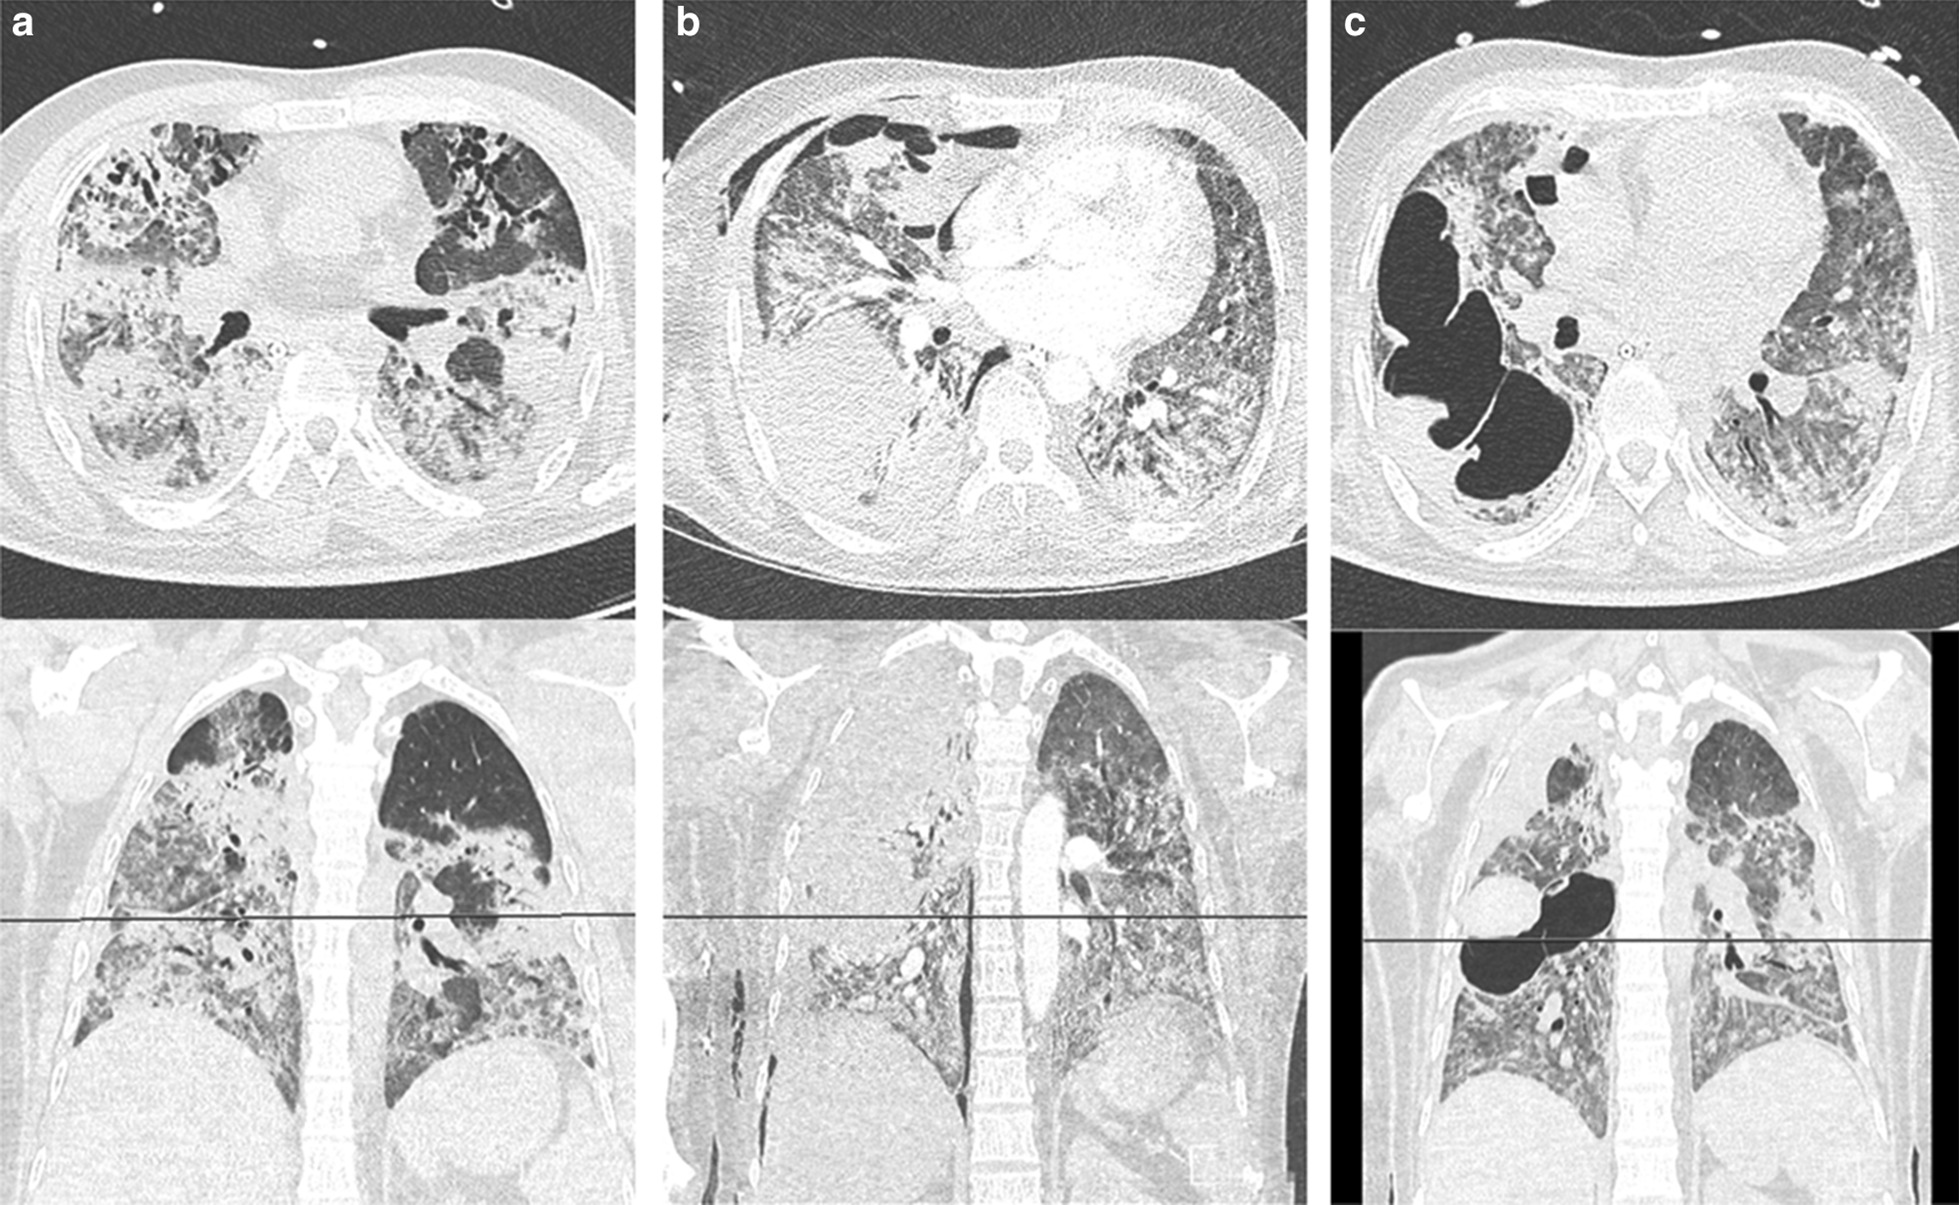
Axial and coronal CT images at form patient 8 on a 5th May 2020, b 26th May 2020, and c 6th June 2020
